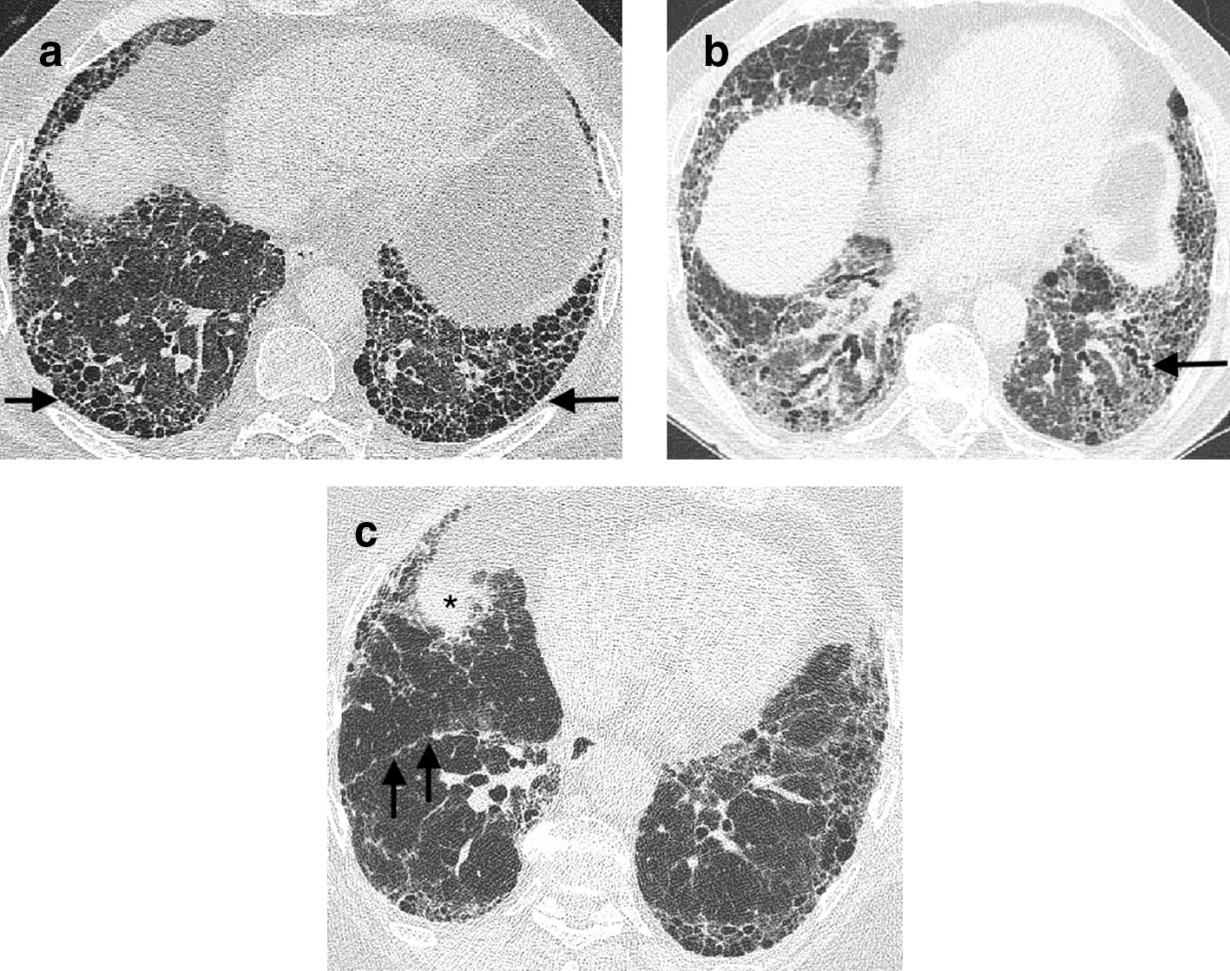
CT signs of lung fibrosis. Honeycomb cysts (arrow) lying in layers in the peripheral basal aspect of the lower lobes (a). Dilated varicose tortuous airways representing traction bronchiectasis (arrow) lying amidst dense fibrotic lung in the lower lobes (b). Volume loss in the lower lobes in a patient with idiopathic pulmonary fibrosis (c). In health, on axial CT images the most inferior aspect of the oblique fissures should reach the anterior chest wall at the level of the hemidiaphragms. However, in this patient, the right oblique fissure (arrows) has been pulled back and now only reaches the midpoint of the lung at a level where the right hemidiaphragm is visible (asterix).
